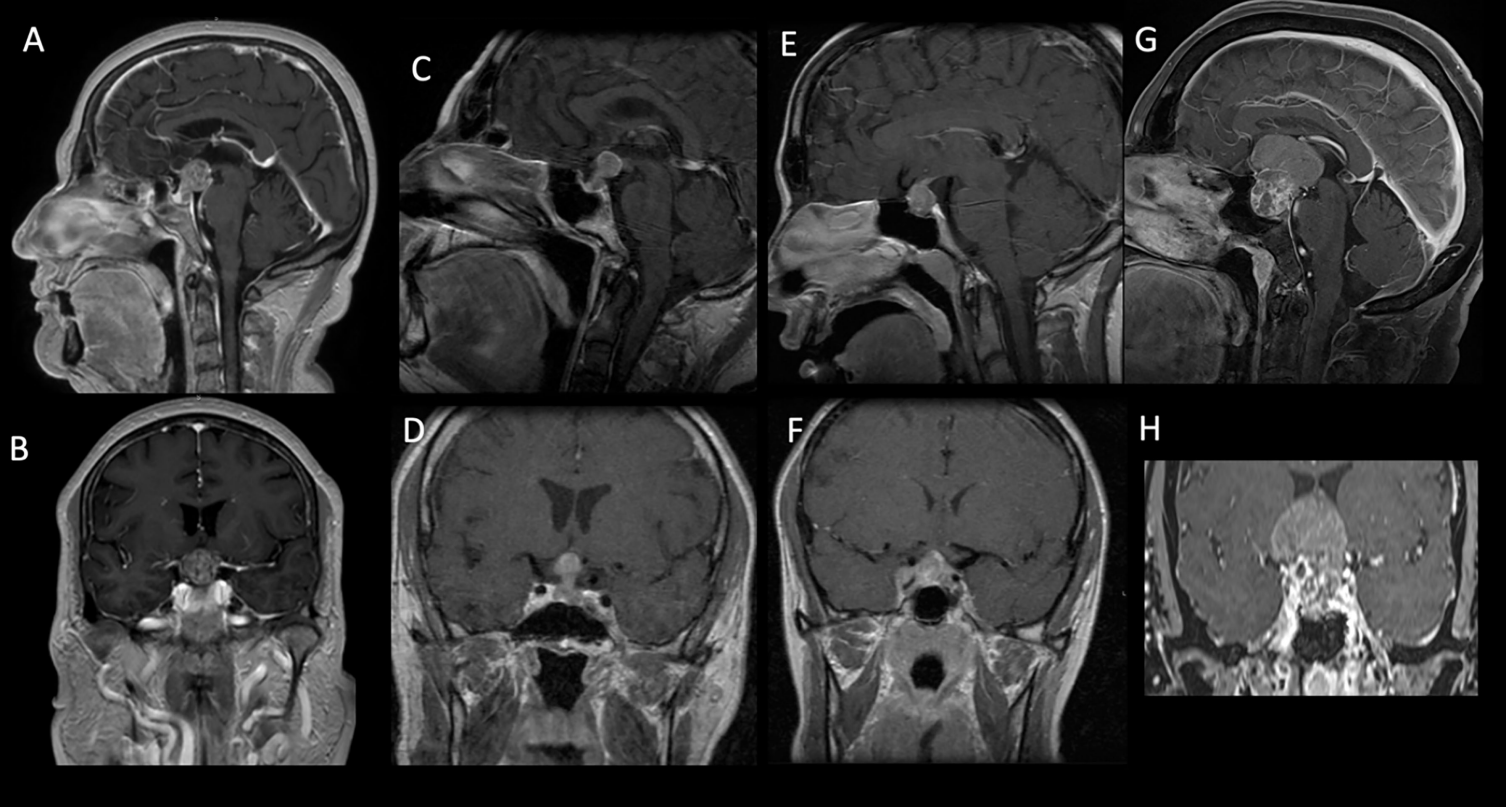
Sagittal and coronal preoperative MRI T1-weighted contrast-enhanced images of patients from our cohort showing intra- and suprasellar lesions with inhomogeneous enhancement. The pathology report disclosed breast adenocarcinoma (A, B), lung adenocarcinoma (C, D), clear cell renal carcinoma (E, F), and uterine cervix carcinoma (G, H).
